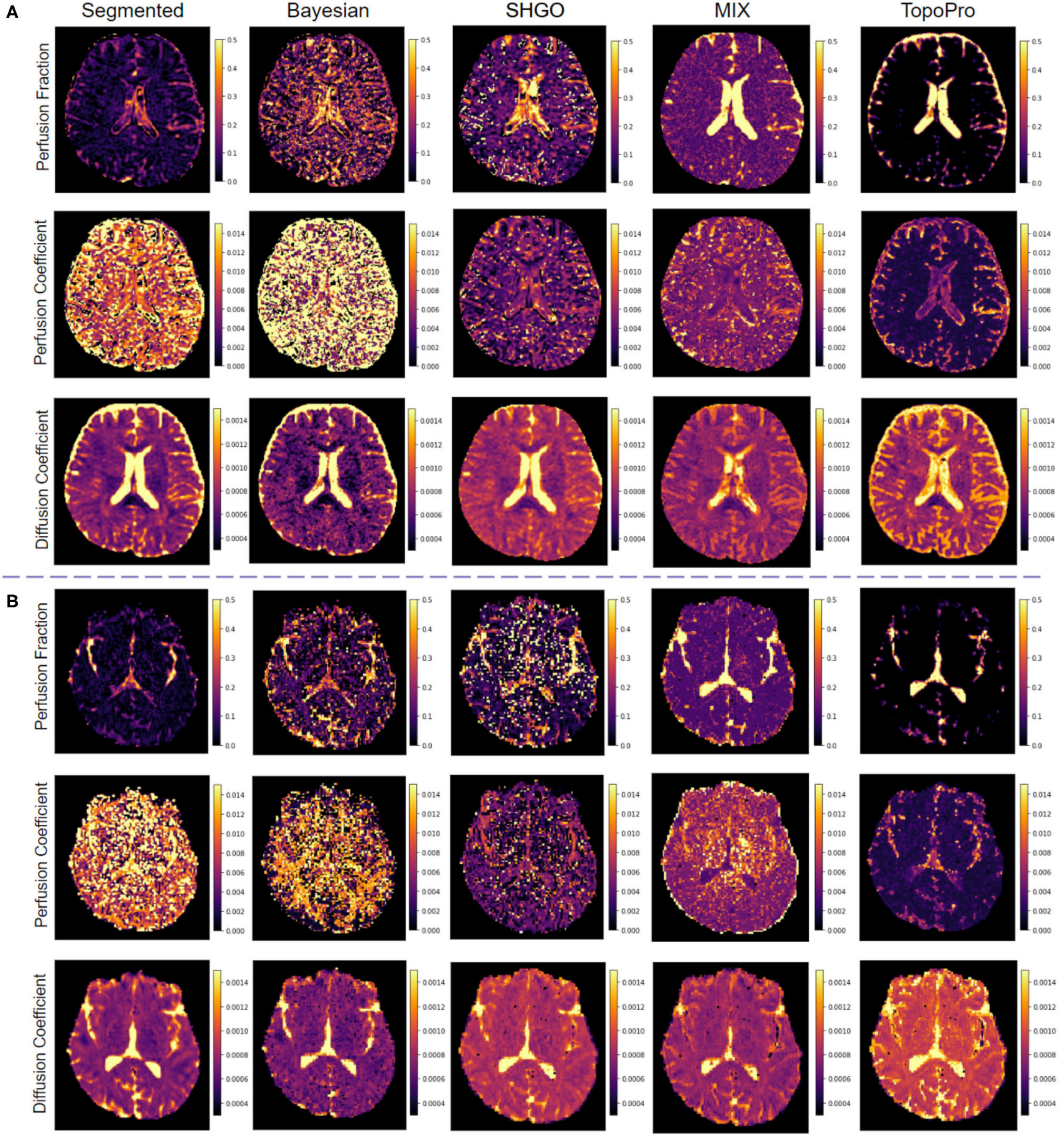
Comparisons of TopoPro on two different real human brain datasets vs. 4 different methods: (A) DWI dataset with dimensions: 256 × 256 × 54 × 21 - slice 33 and (B) DWI dataset with dimensions: 140 × 140 × 84 × 49 - slice 42. Notice how TopoPro provides improved estimates of the different parameters of the IVIM model in both data. The results are compared against methods: Segmented, Bayesian, SHGO, and MIX.
